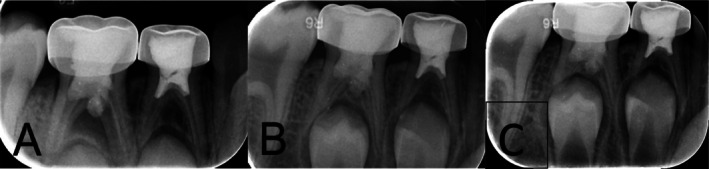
Radiographic healing progression. (A) Three‐month follow‐up radiograph demonstrating initial trabecular bone regeneration in the furcation area. (B) Twelve‐month follow‐up radiograph showing complete resolution of furcal radiolucency with trabecular bone fill, re‐establishment of lamina dura, and osseous integration of the overextended CEM cement. (C) Eighteen‐month follow‐up radiograph confirming continued trabecular bone maturation, complete lamina dura reformation, and stable integration of the extruded CEM cement without any signs of adverse tissue response.
